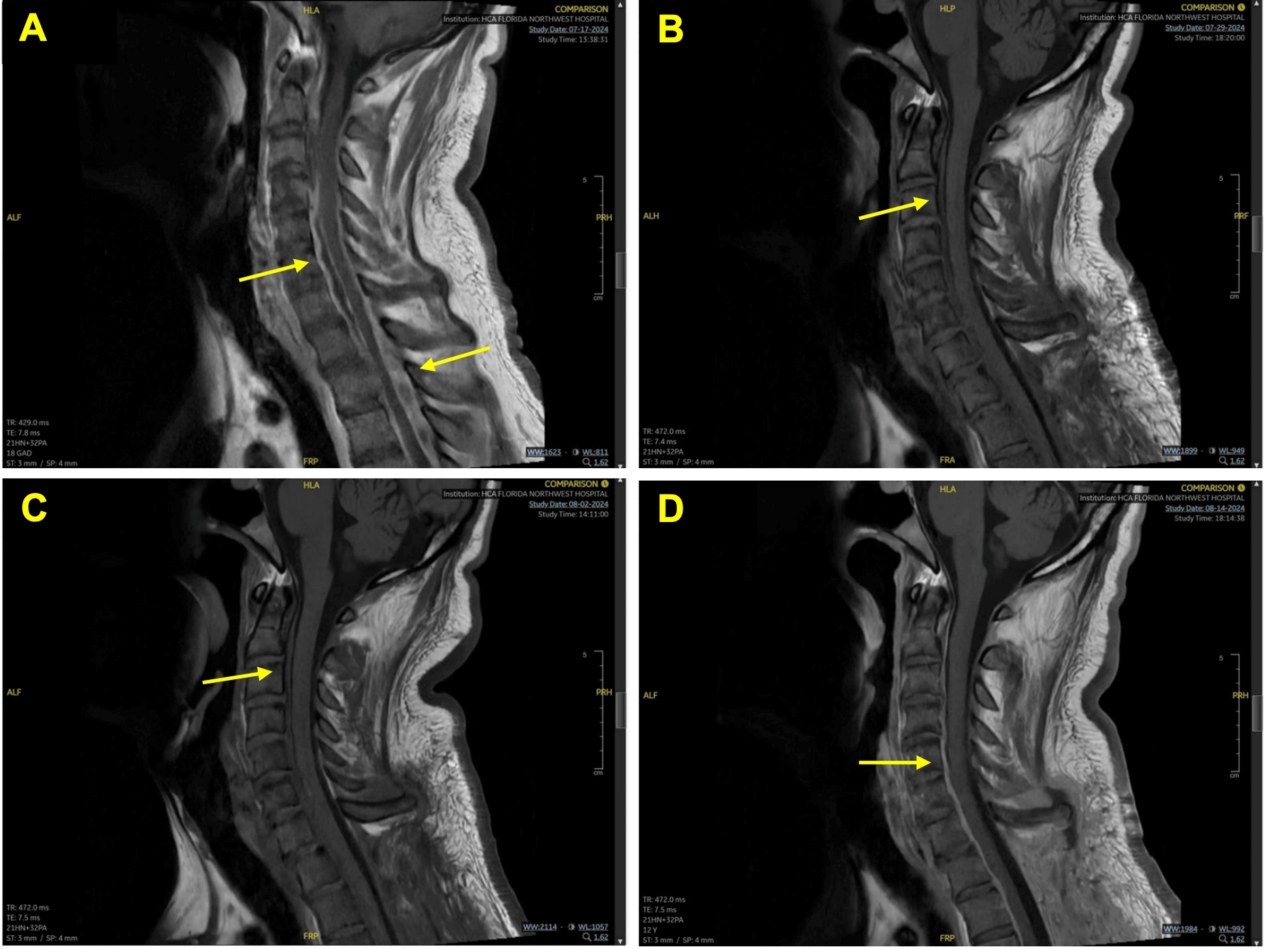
Cervical magnetic resonance imaging (MRI) (A) Initial cervical MRI revealing ventral C2-T1 spinal epidural abscess and dorsal T1-T4 spinal epidural abscess. (B) Post T1-T3 laminectomy MRI of the cervical spine. (C and D) Interim period MRI demonstrating persistent ventral spinal epidural abscess
